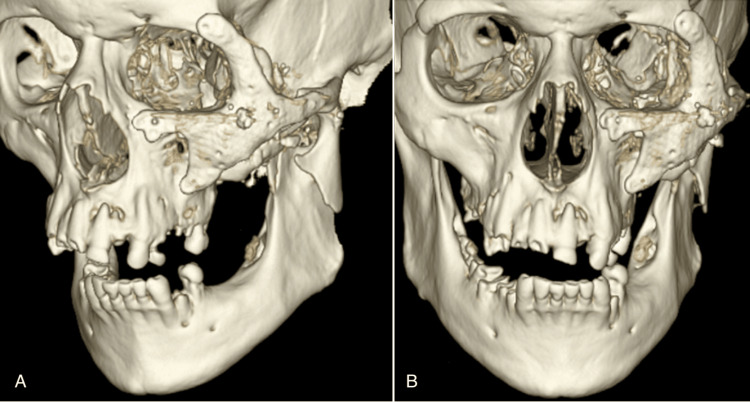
Postoperative day one 3D CT images show closed fixation with the placement of a titanium implant. Left oblique (A) and frontal (B) views are shown.
